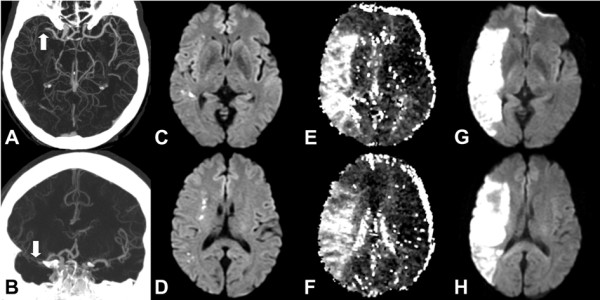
61 year old male presenting with left facial droop and dysarthria. Initial imaging studies were performed 6 hours after witnessed onset of speech difficulties. However, patient noted symptoms suggestive of facial weakness 12 hours prior to imaging. A &B. CTA demonstrates a proximal right MCA occlusion (white arrows). C &D. Initial DWI shows multiple punctate foci of diffusion abnormality consistent with a small acute infarct. E &F. Time to peak (TTP) MR perfusion map demonstrates a large volume of underperfused area involving the majority of the right MCA territory and consequently a large diffusion/perfusion mismatch. The patient did not undergo thrombolytic therapy because of the uncertain time between stroke onset and presentation. G &H. Follow-up MRI shows an infarction involving the majority of the right MCA territory. Whole brain map volume measurements for this patient are: DWI: 2.1 ml; MTT: 117 ml (mismatch: 115.4 ml); TTP: 28 ml (mismatch 25.9 ml).
